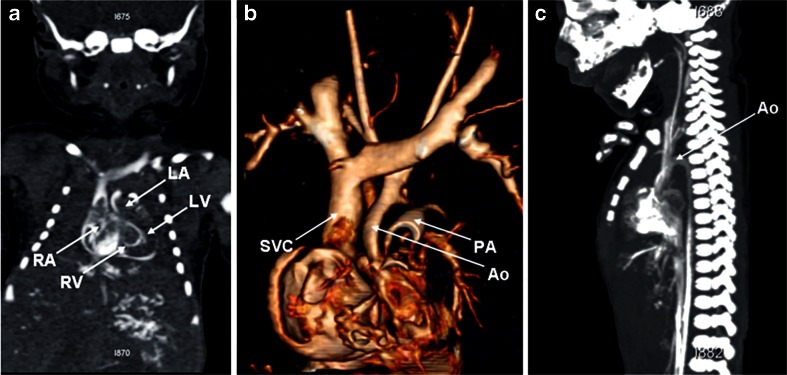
Postmortem CT angiography of the heart in a 37-week foetus with normal cardiac anatomy. a A four-chamber view, (b) reconstructed 3-D view of the great vessels, and (c) aortic arch are demonstrated. *Ao* aorta, *LA* left atrium, *LV* left ventricle, *PA* pulmonary artery, *RA* right atrium, *RV* right ventricle, *SVC* superior vena cava. Reproduced with permission [29]
